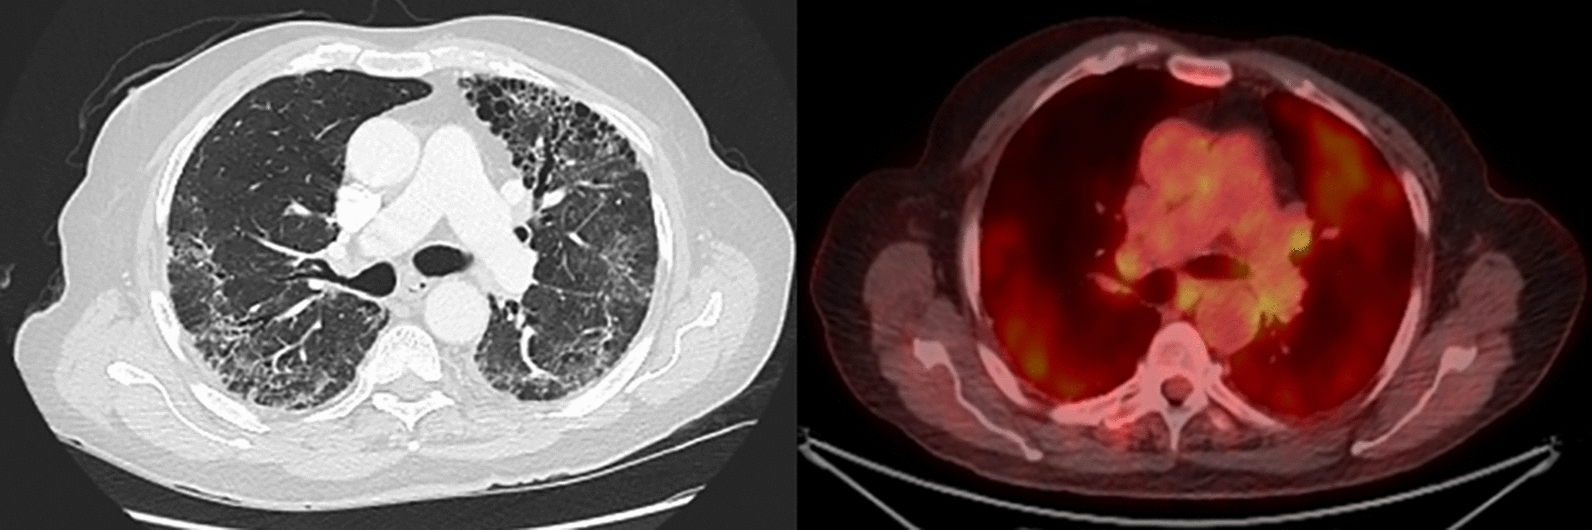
72-year-old man with pulmonary fibrosis and dyspnea on exertion. Axial CT image and fused axial FDG PET/CT demonstrating areas of bilateral heterogeneous FDG uptake, corresponding to honeycombing and reticular opacities
